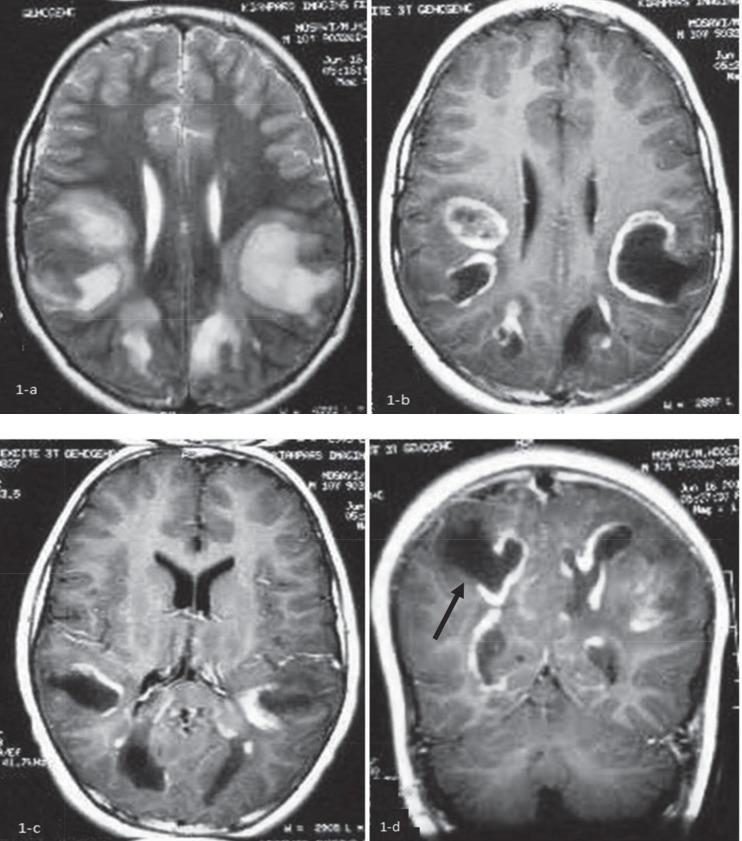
Initial brain MRI without contrast shows bilateral abnormal high signal lesions in the subcortical and deep posterior white matter in T2-WI sequence, almost isointense with CSF in the center, surrounded by a peripheral halo (a): After contrast injection In T1-WI images, low signal center with peripheral complete and incomplete ring enhancement is seen, suggesting necrotic or tumefactive center (b, c, d). Some cortical extension of the lesion in the right posterior parietal lobe is seen (Black arrow in d
